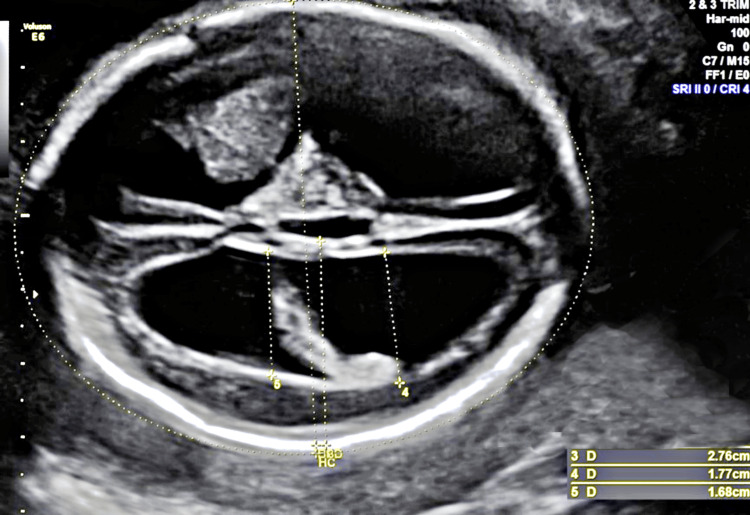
Ultrasound of the fetal head depicting ventriculomegaly.
